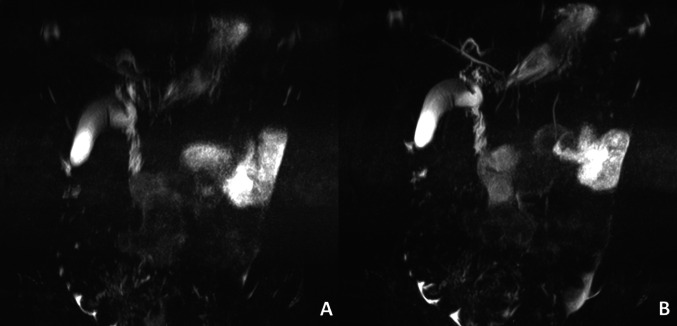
3D T2-weighted MRI sequences of the same patient. A represents the 3D T2-weighted MRI sequence from an MRCP exam without the use of the respiratory trigger technique. B represents the 3D T2-weighted MRI sequence from an MRCP exam with the use of the respiratory trigger technique. Notice the detailed view of biliary and pancreatic ducts on B in comparison to blurry views on A
